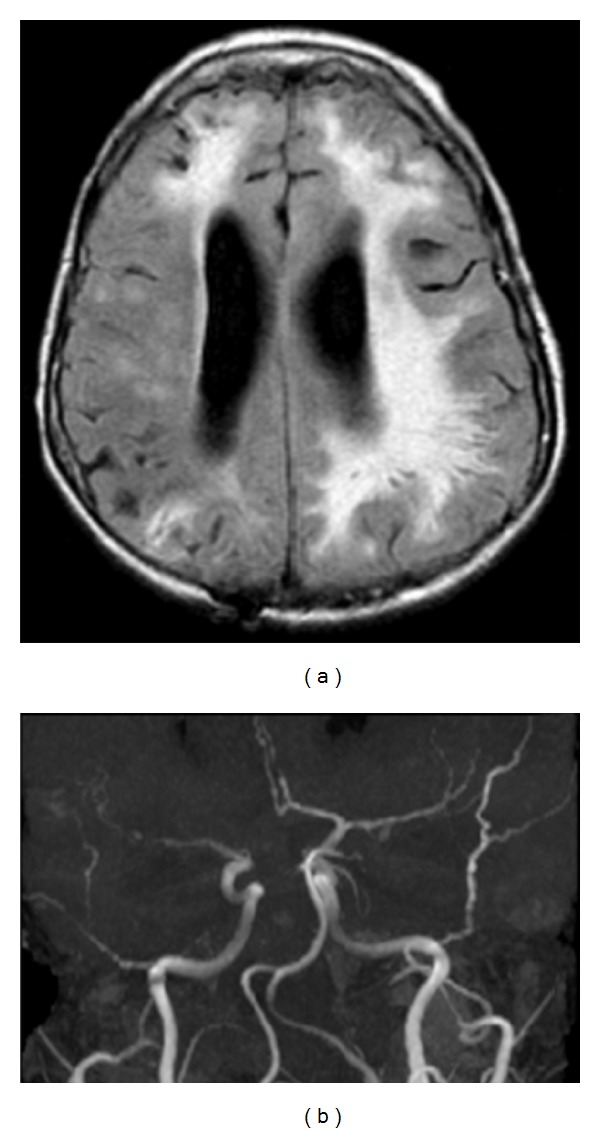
(a) Three mm axial fluid attenuated inversion recovery (FLAIR) brain magnetic resonance images (MRIs) demonstrating extensive subcortical white matter hyperintensity. (b) Magnetic resonance angiogram (MRA) demonstrating beading of small and medium intracranial vessels.
